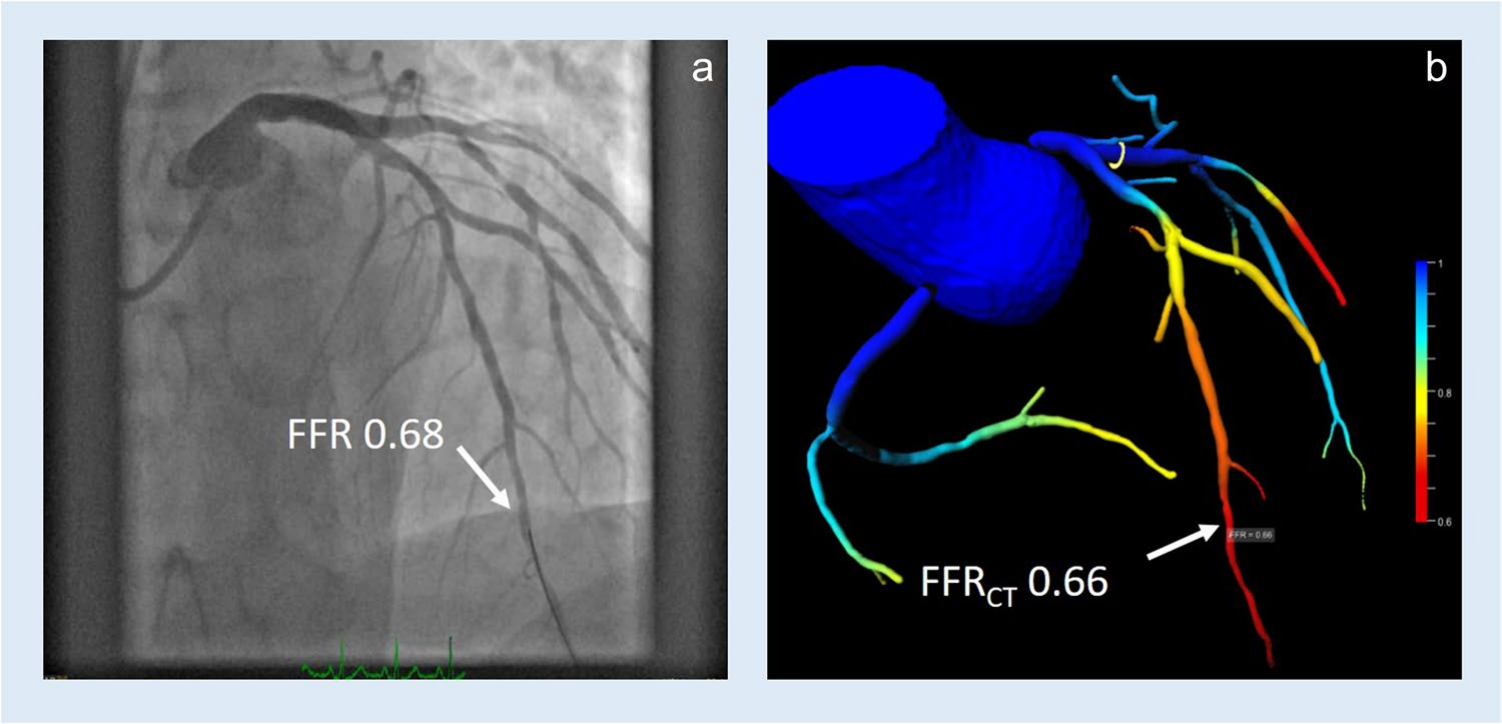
a Invasive angiogram with an FFR wire placed in the distal left anterior descending artery. b 3D CT reconstruction of the coronary tree showing FFR_CT_
